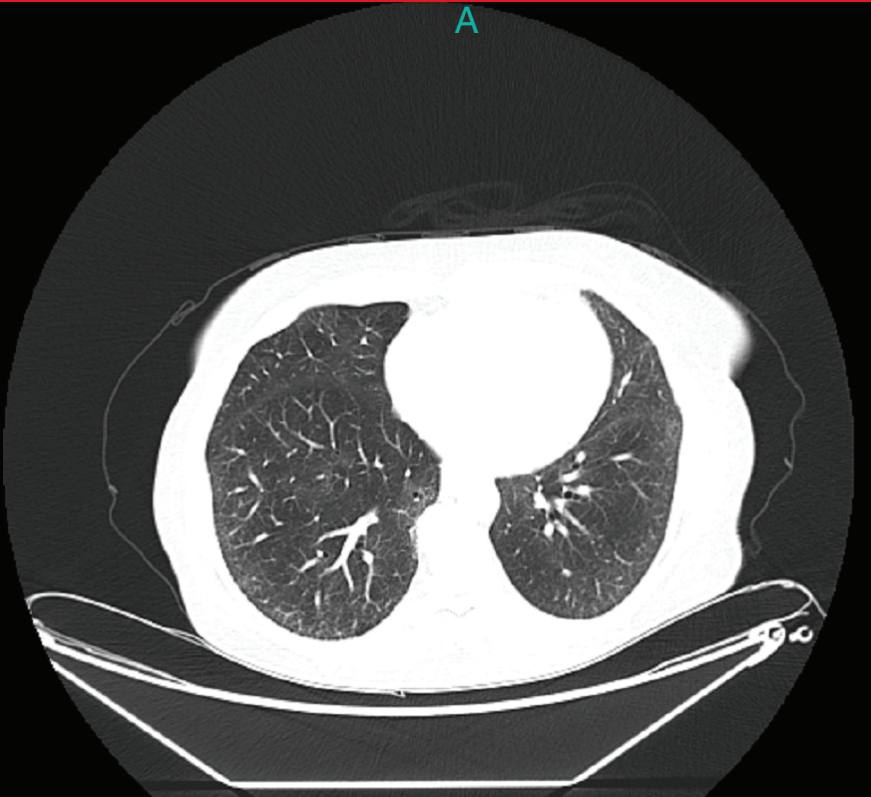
Follow-up chest CT performed 1 month after COVID-19 diagnosis showing almost complete resolution of prior lung infiltrates.
